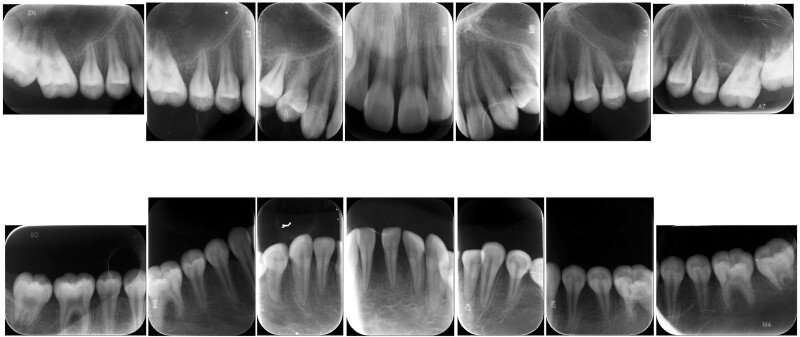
Periapical radiographs showing alveolar bone loss at the first molars and incisors.
